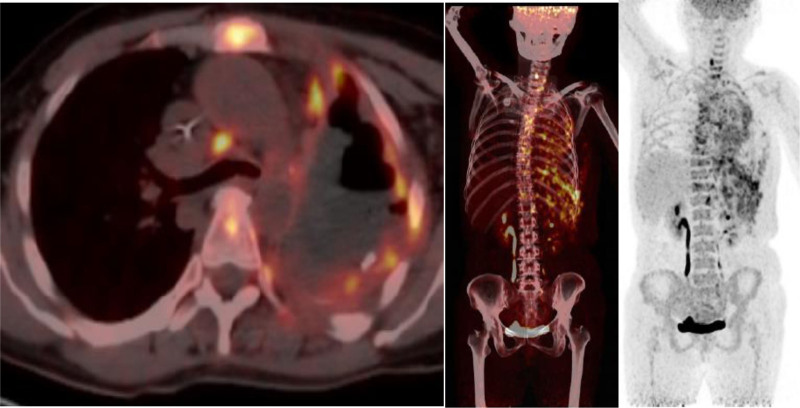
Above is the second 18F-FDG PET/CT images, which show that the lesion has invaded multiple tissues. 18F-FDG PET/CT = ^18^F-fluorodeoxyglucose positron emission tomography/computed tomography.
